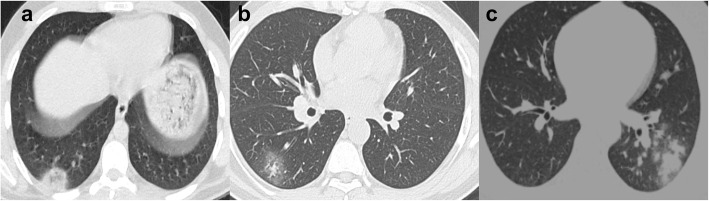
Axial chest CT lung window in different cases with COVID-19 pneumonia showing a the reversed halo sign: right lower lobe posterior basal segment patchy consolidation with internal lucency. b Halo sign: patchy consolidation with a surrounding halo of ground-glass density at the right lower lobe apical segment (b) and at the left lower lobe (c)
